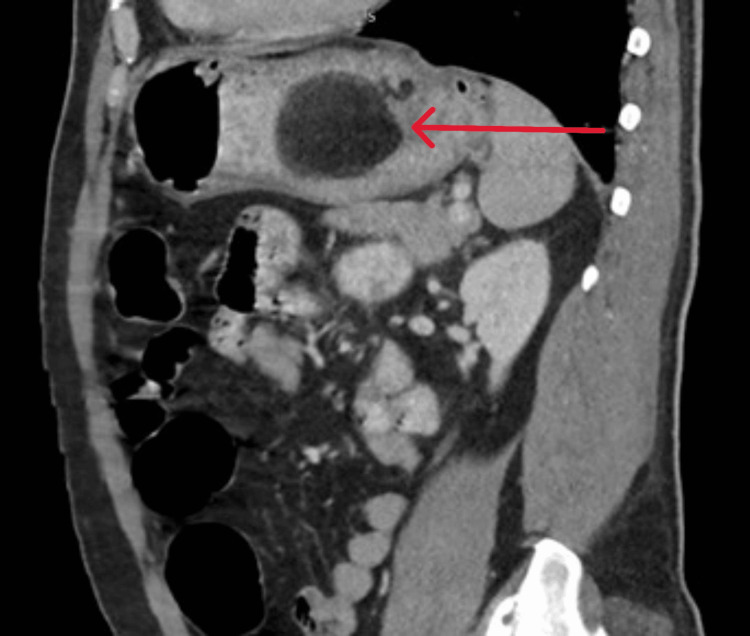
CT scan-sagittal view, fat-containing intramural subepithelial mass lesion CT scan (sagittal view), another view of the gastric mass (red arrow)
